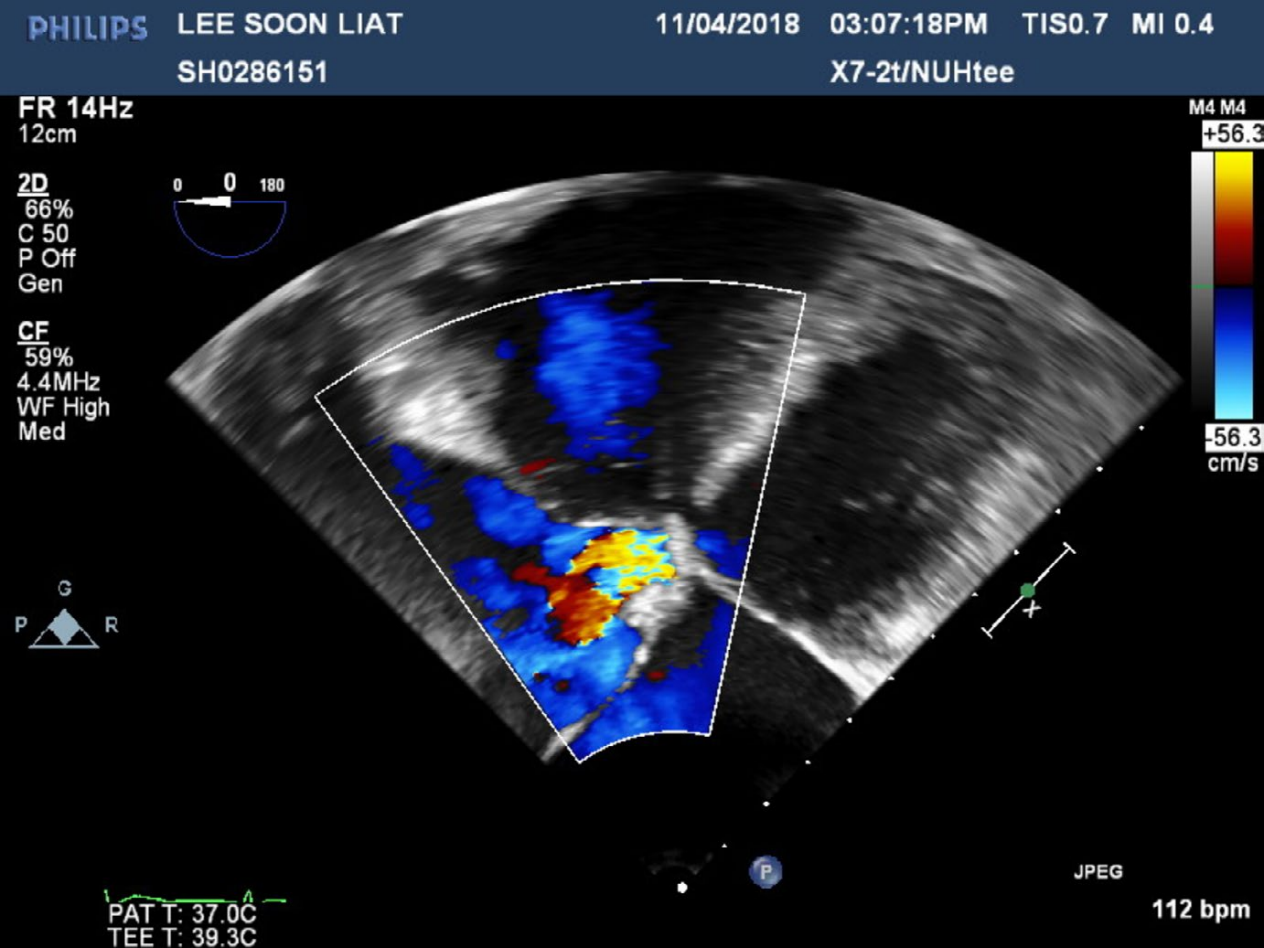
Preoperative Transesophageal Echocardiogram showing TR
